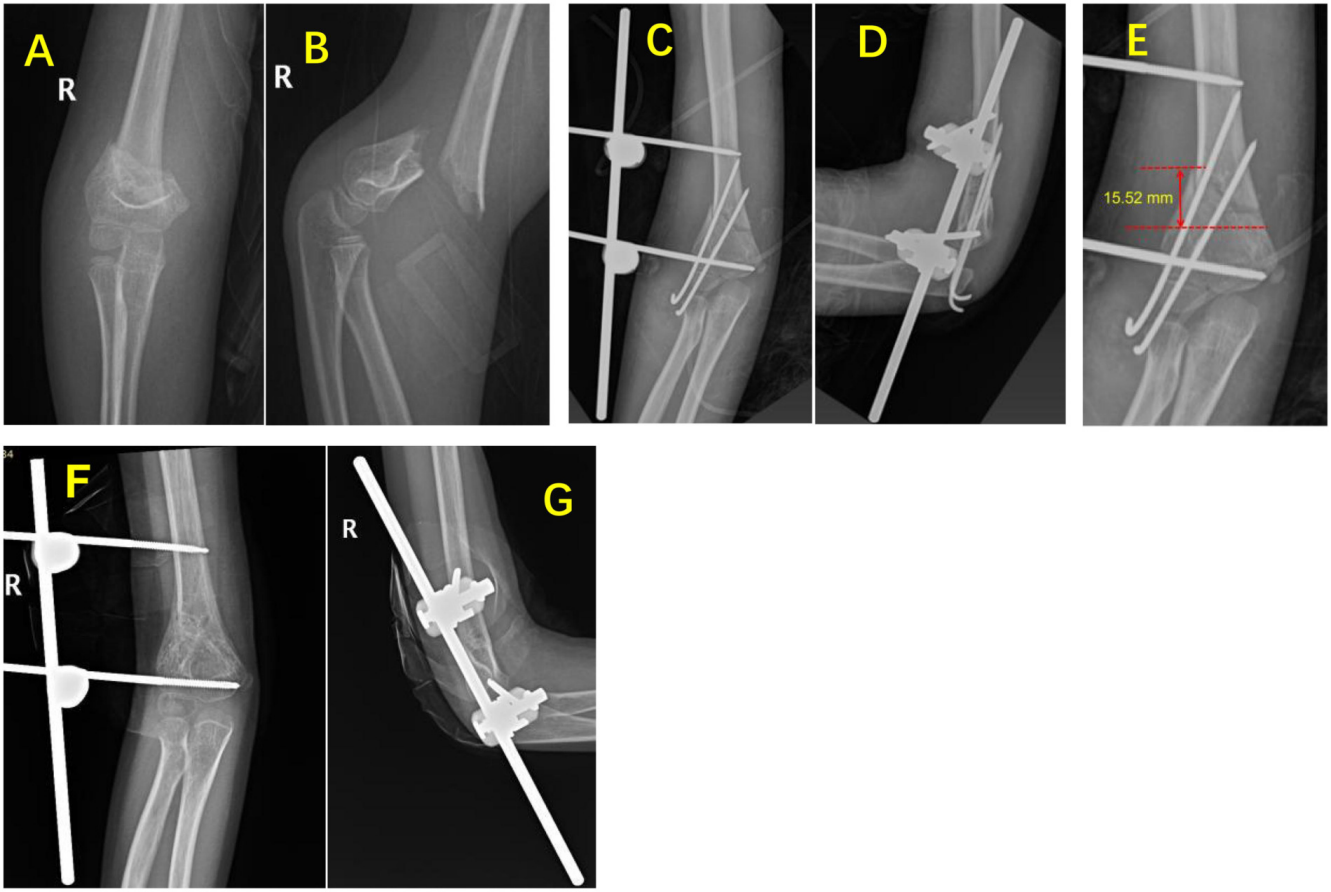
Representative case of linear configuration external fixation. A 6-year-11-month-old girl with right distal humeral MDJ fracture (oblique type). (A,B) Preoperative anteroposterior and lateral radiographs showing displaced fracture. (C,D) Intraoperative radiographs after closed reduction with linear external fixation plus K-wire augmentation. (E) Fracture line height measurement: 15.52 mm. (F,G) 12-week follow-up radiographs demonstrating satisfactory union; external fixator removed.
